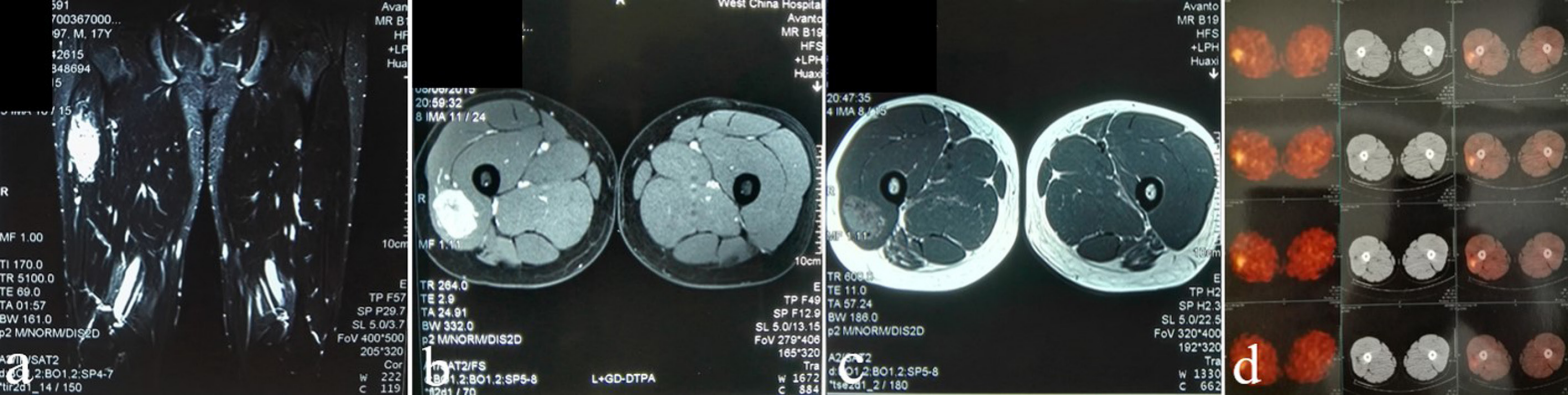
MRI and PET scans A soft tissue is shown in the upper right thigh, with long T1 and T2 signals (a-c). PET scan showing a soft tissue mass with a maximum SUV score of 2.6 (d).
